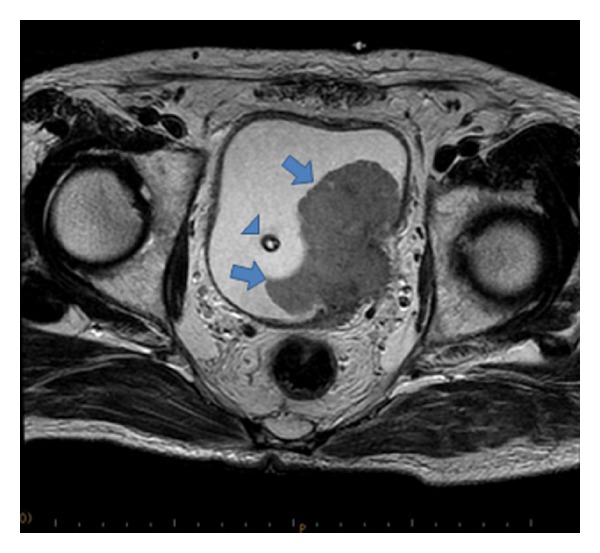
Enhanced MRI showing a bladder tumor at the left wall (arrow). Arrow head shows urethral balloon catheter.
